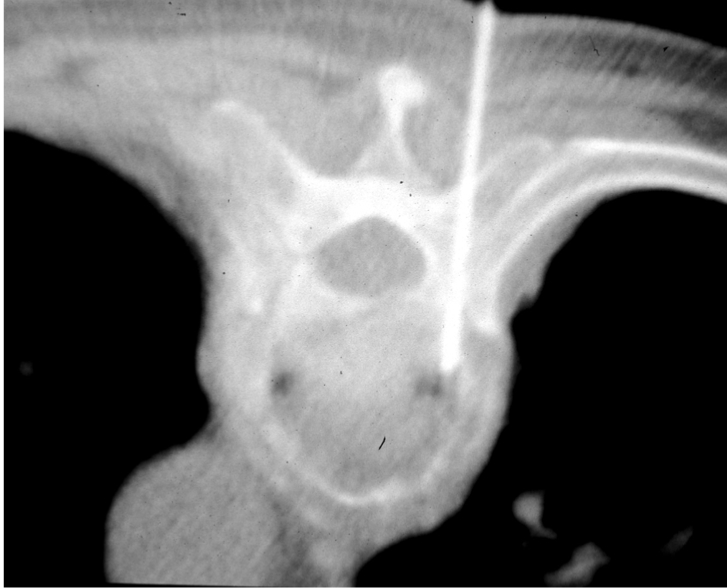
Biopsy of the T8 vertebra using the transpedicular approach in a 73-year-old man with metastasis. CT fluoroscopic image taken with the patient lying prone shows the tip of the Ostycut needle within the right side of T8 vertebral body.
